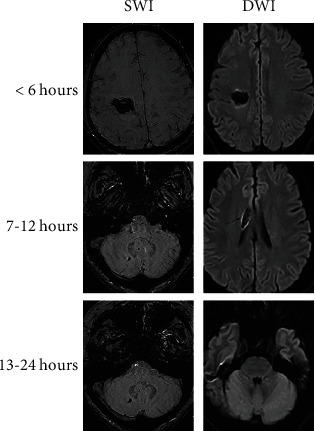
Image characteristics of DWI and SWI at three different periods since intracerebral hemorrhage the onset. DWI, diffusion-weighted imaging; SWI, susceptibility-weighted imaging.
